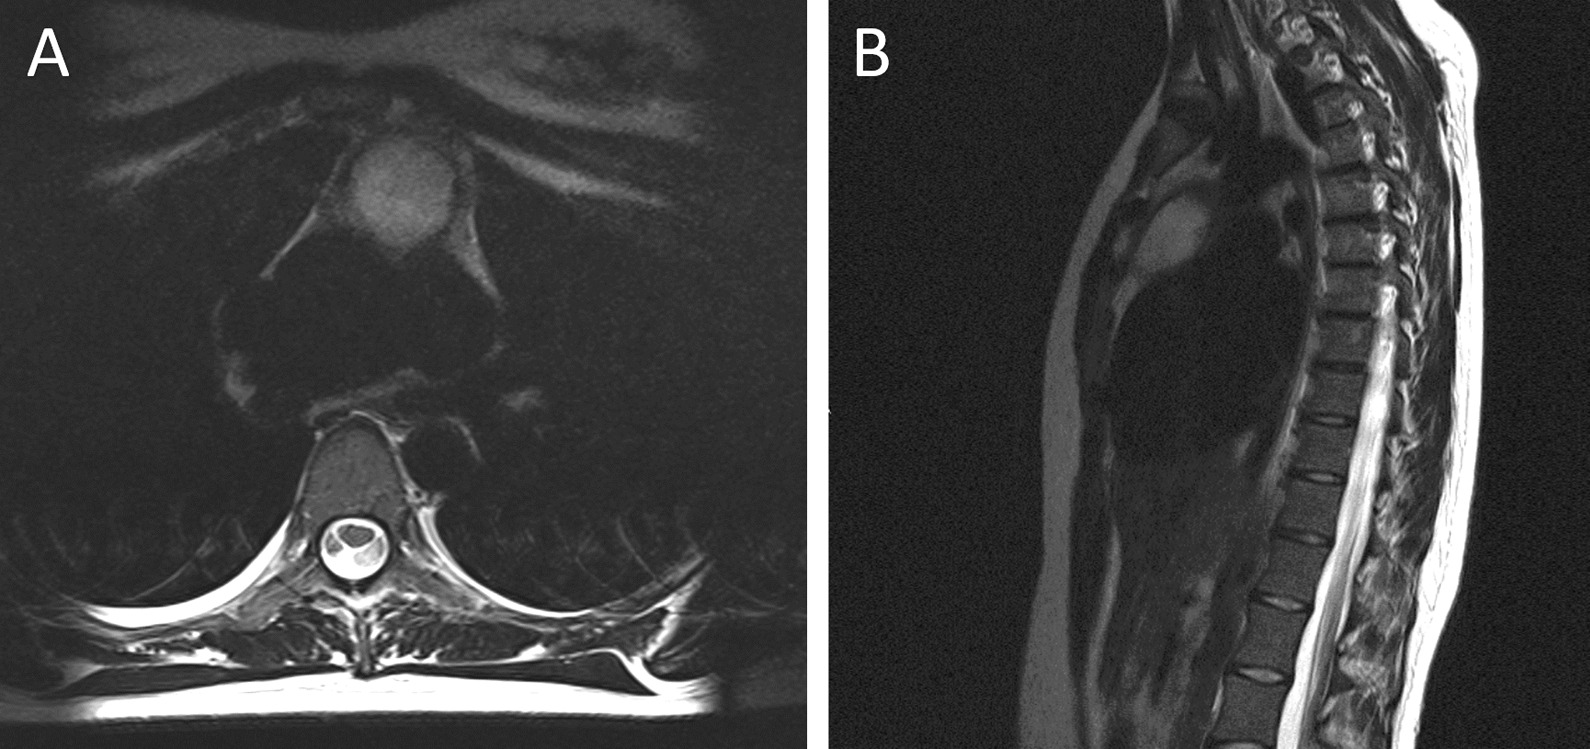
Magnetic resonance imaging (MRI) demonstrating anterior mediastinal mass (A) Transverse T2 image showing a heterogeneous anterior mediastinal mass. B Sagittal fat suppressed image demonstrating anterior mediastinal mass
